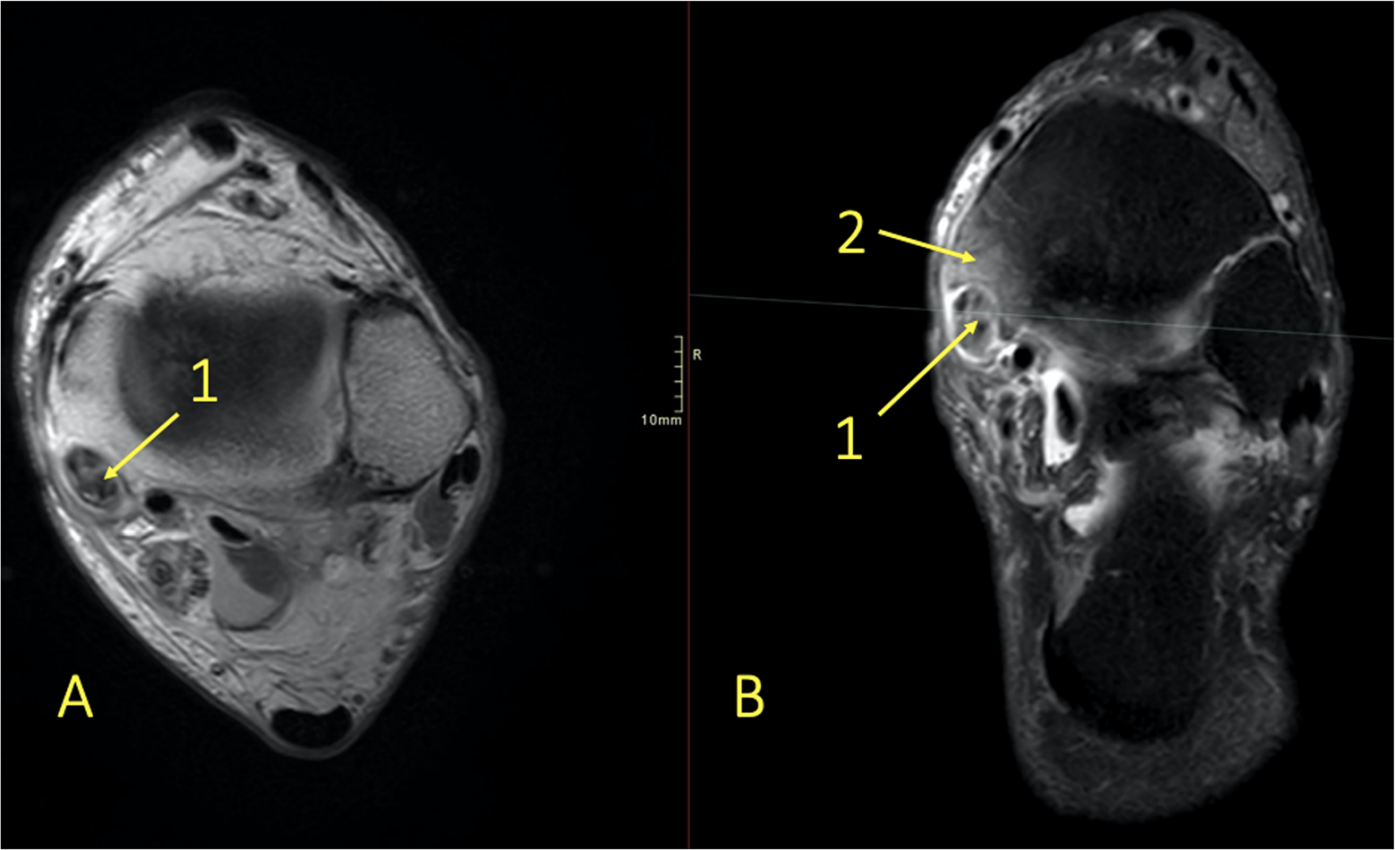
A 65-year-old male with chronic ankle pain after ankle trauma about 3 weeks earlier and a suspected rupture of the tibialis posterior tendon. MRI showed tendinopathy. (1) A partial rupture of the tibialis posterior tendon and (2) BME in the medial malleolus were visible
